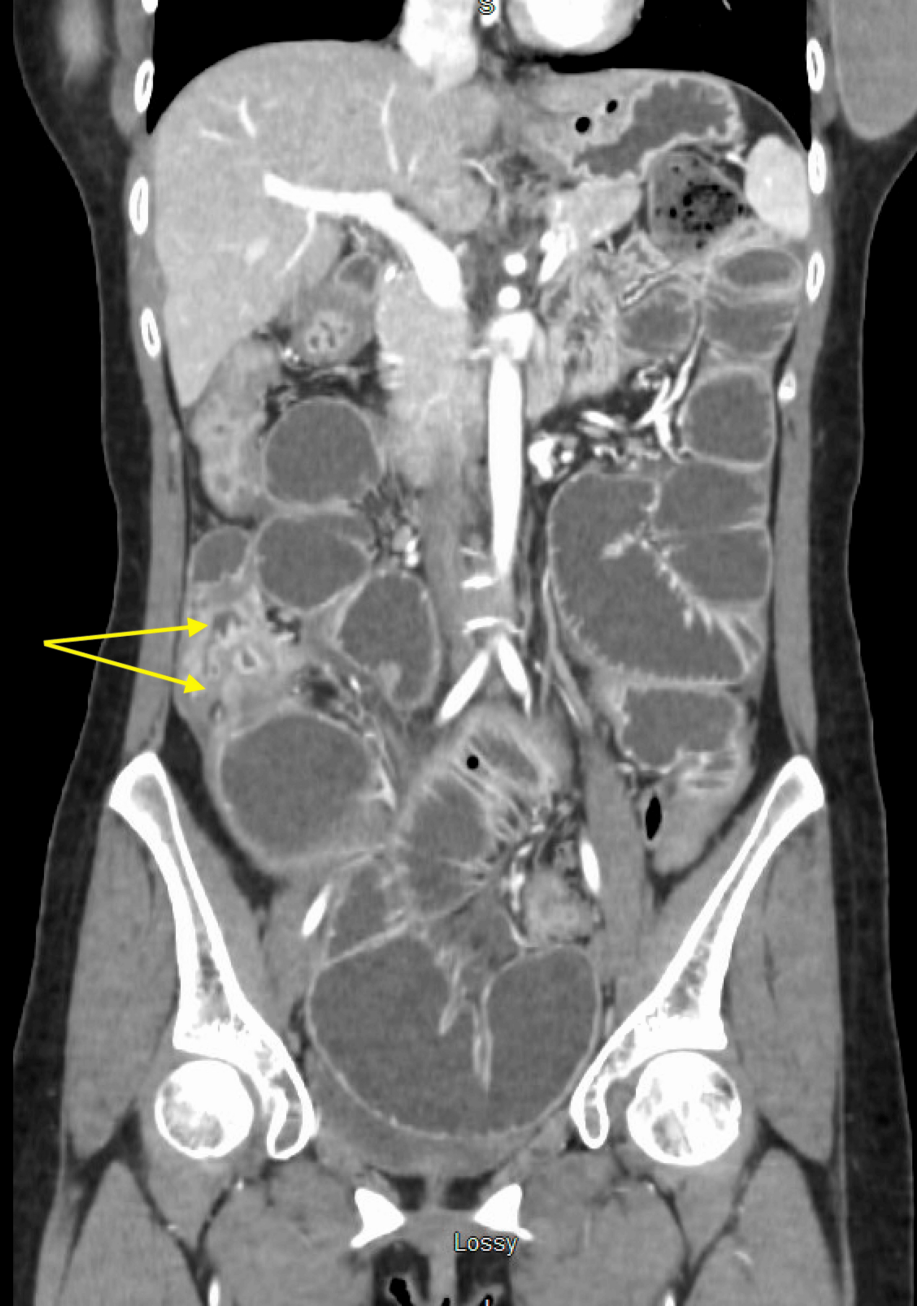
Coronal view of CT abdomen and pelvis demonstrating inflammatory change
